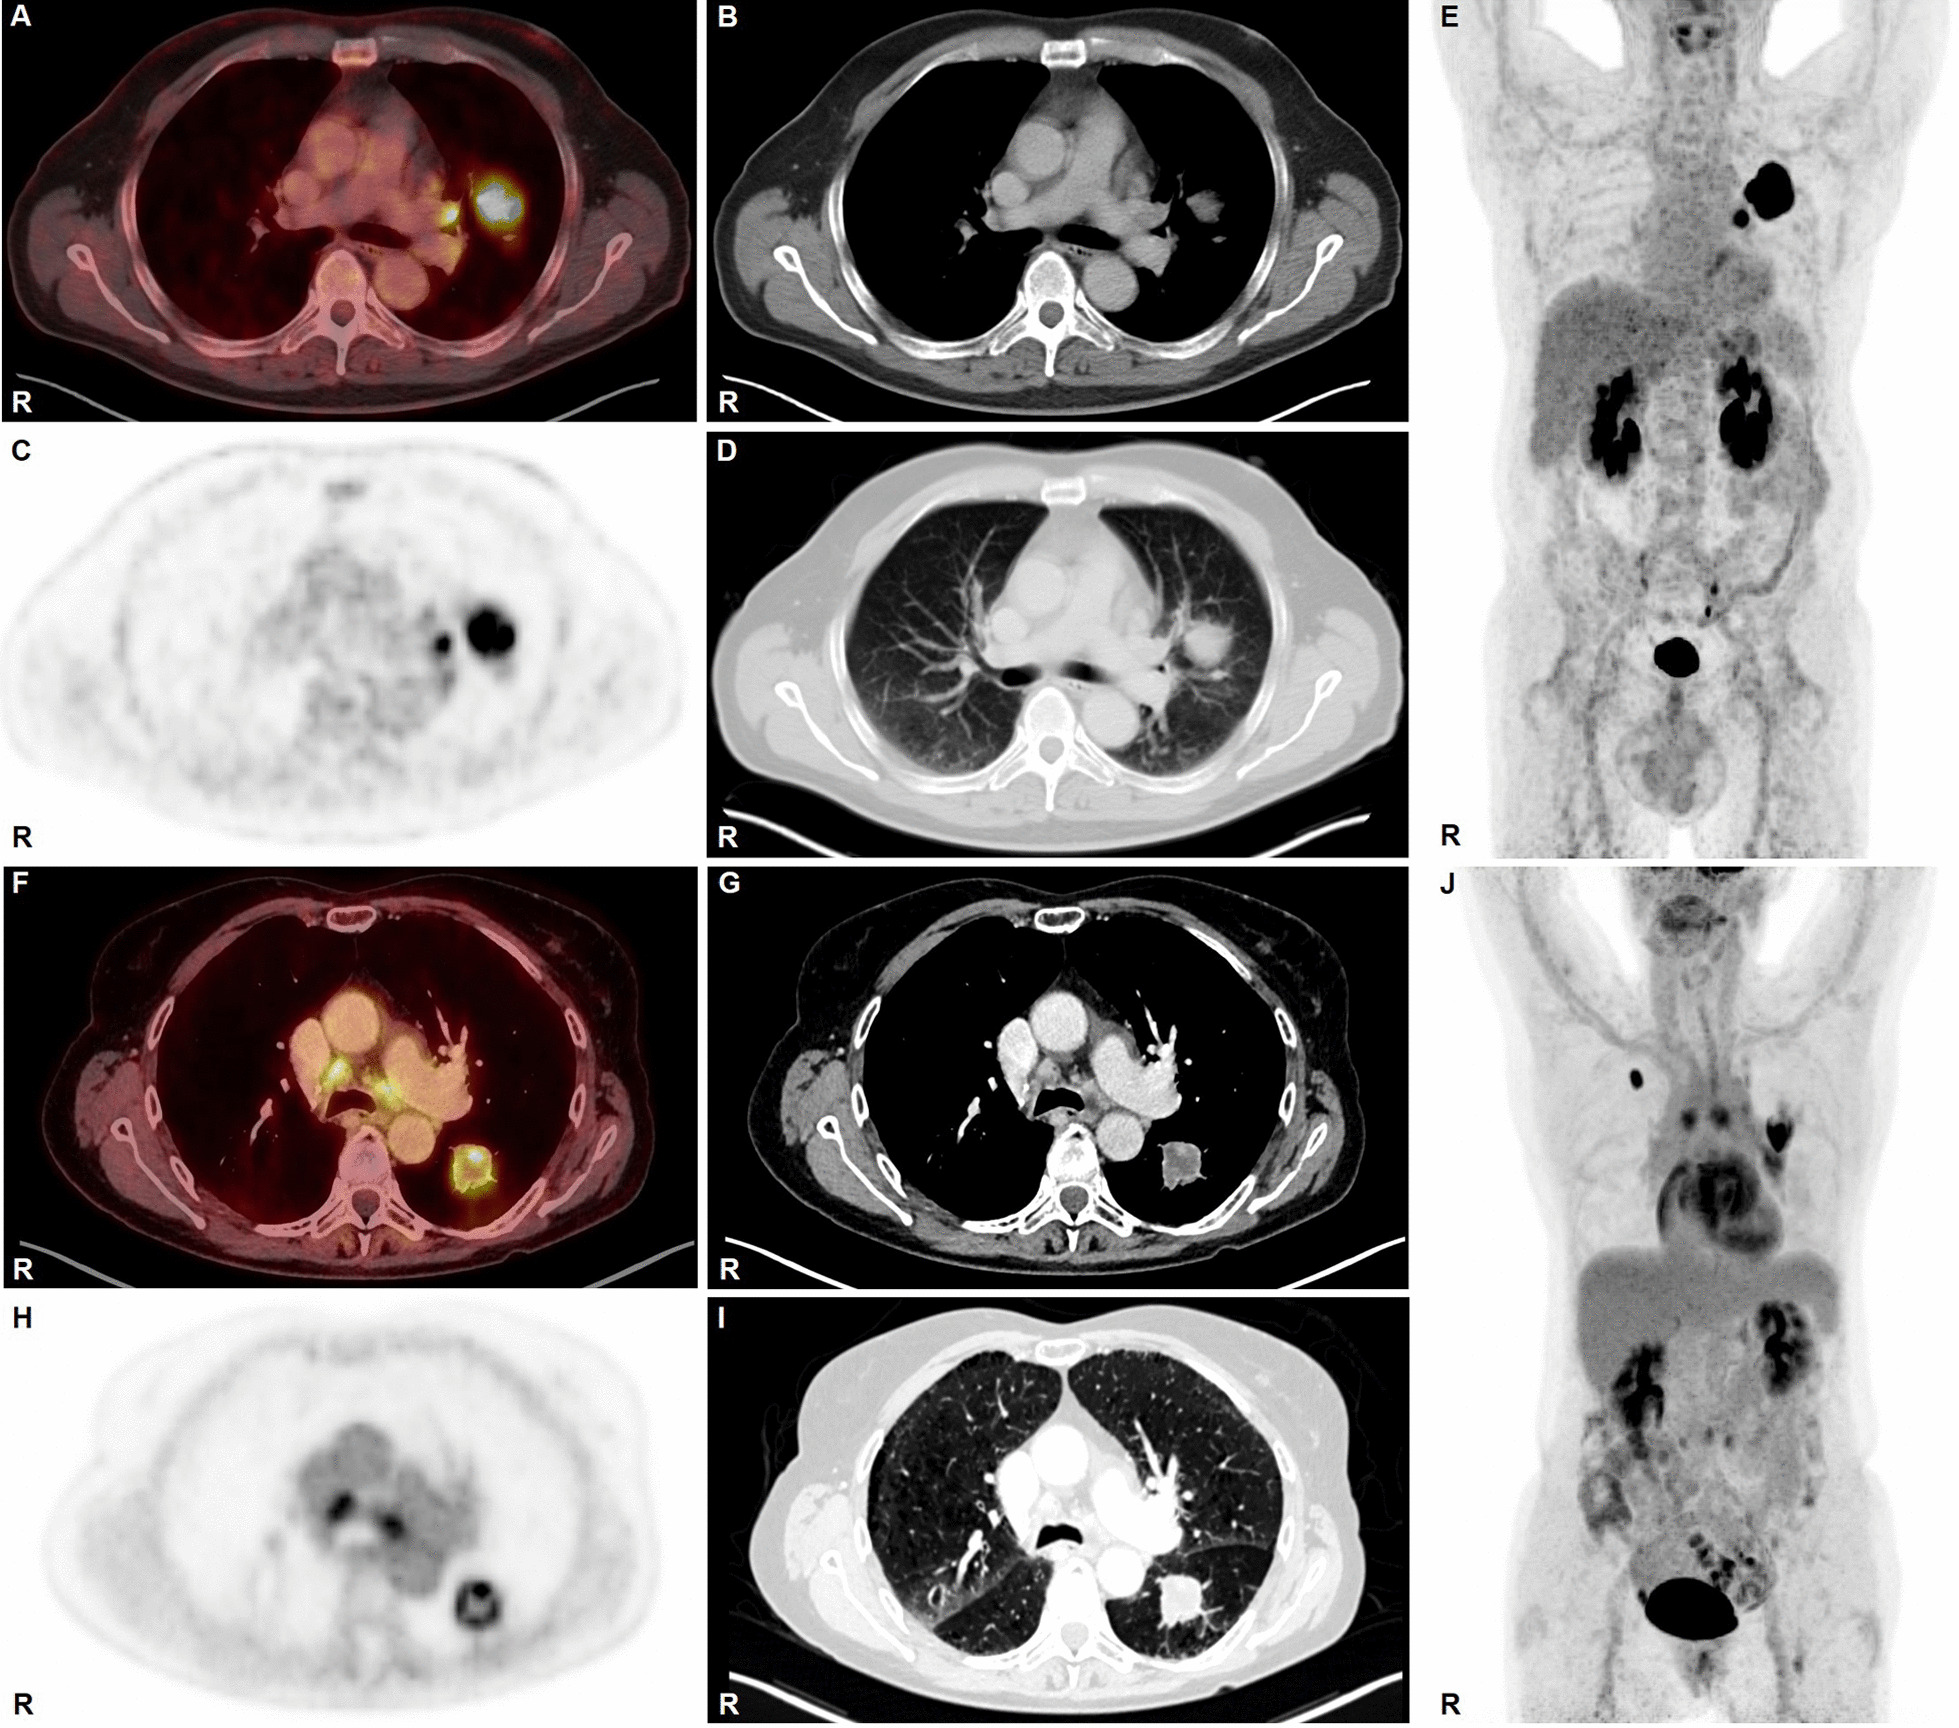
A–E true positive lymph node: [^18^F]FDG PET/CT: male, age 58 years, LCNEC pT3 pN1 (1/11) M0, stage IIIa. SUV_max_ primary 17.7, SUV_max_ true positive lymph node metastasis 10.8. A [^18^F]FDG PET/CT fusion, B CT chest soft tissue window, C PET attenuation-corrected emission, D CT chest lung window, E PET maximum intensity projection (MIP). F–J false positive lymph node: [^18^F]FDG PET/CT: female, age 66 years, LCNEC pT1c pN0 (0/21) pM1a (resected single metastasis in contralateral lung), stage IVa. Patient underwent EBUS-TBNA 3 days prior to FDG PET/CT. SUV_max_ primary 15.8, SUV_max_ false positive lymph nodes 5.2. F [^18^F]FDG PET/CT fusion, G CT chest soft tissue window, H PET attenuation-corrected emission, I CT chest lung window, J PET maximum intensity projection (MIP). Last follow-up 7 months after initial diagnosis: no evidence of recurrence
